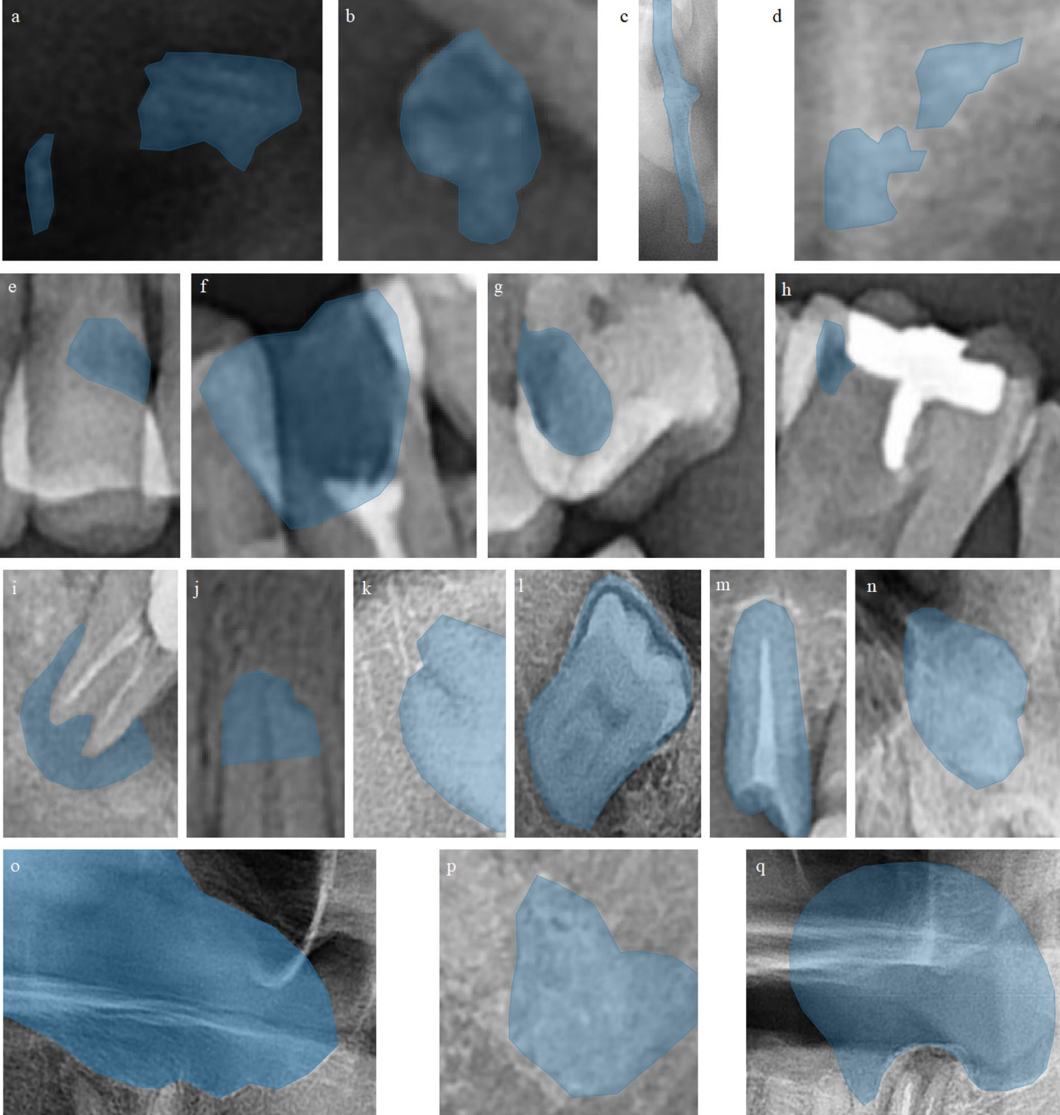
Examples of labeled 17 dental anomalies. (a) Calcified carotid atherosclerotic plaque, (b) lymph node calcification, (c) ossification of stylohyoid ligament, (d) tonsillar calcification, (e) cervical caries or abrasion, (f) dental caries or coronal defect, (g) proximal caries, (h) secondary caries, (i) periapical radiolucency, (j) external root resorption, (k) tooth overlapped with mandibular canal, (l) impacted tooth, (m) residual root, (n) supernumerary tooth, (o) mucosal thickening on maxillary sinus, (p) radiopacity in jaw, and (q) retention pseudocyst on maxillary sinus.
